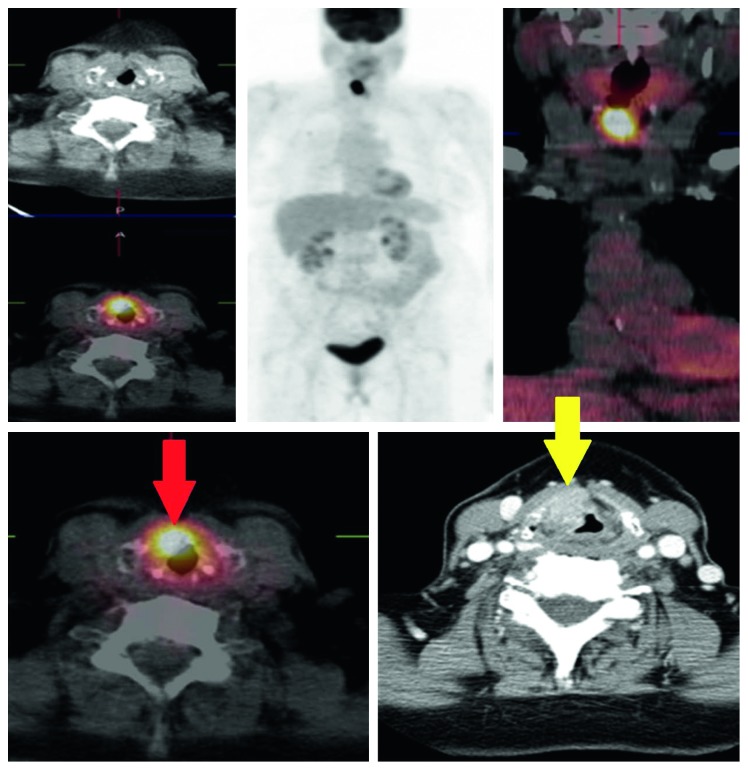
Pt T3 (no cartilage infiltration): PET/CT positive (FP, red arrow) vs. CECT positive (FP, yellow arrow). SUVmax, 19.99; MTV, 8.81 cm^3^.
